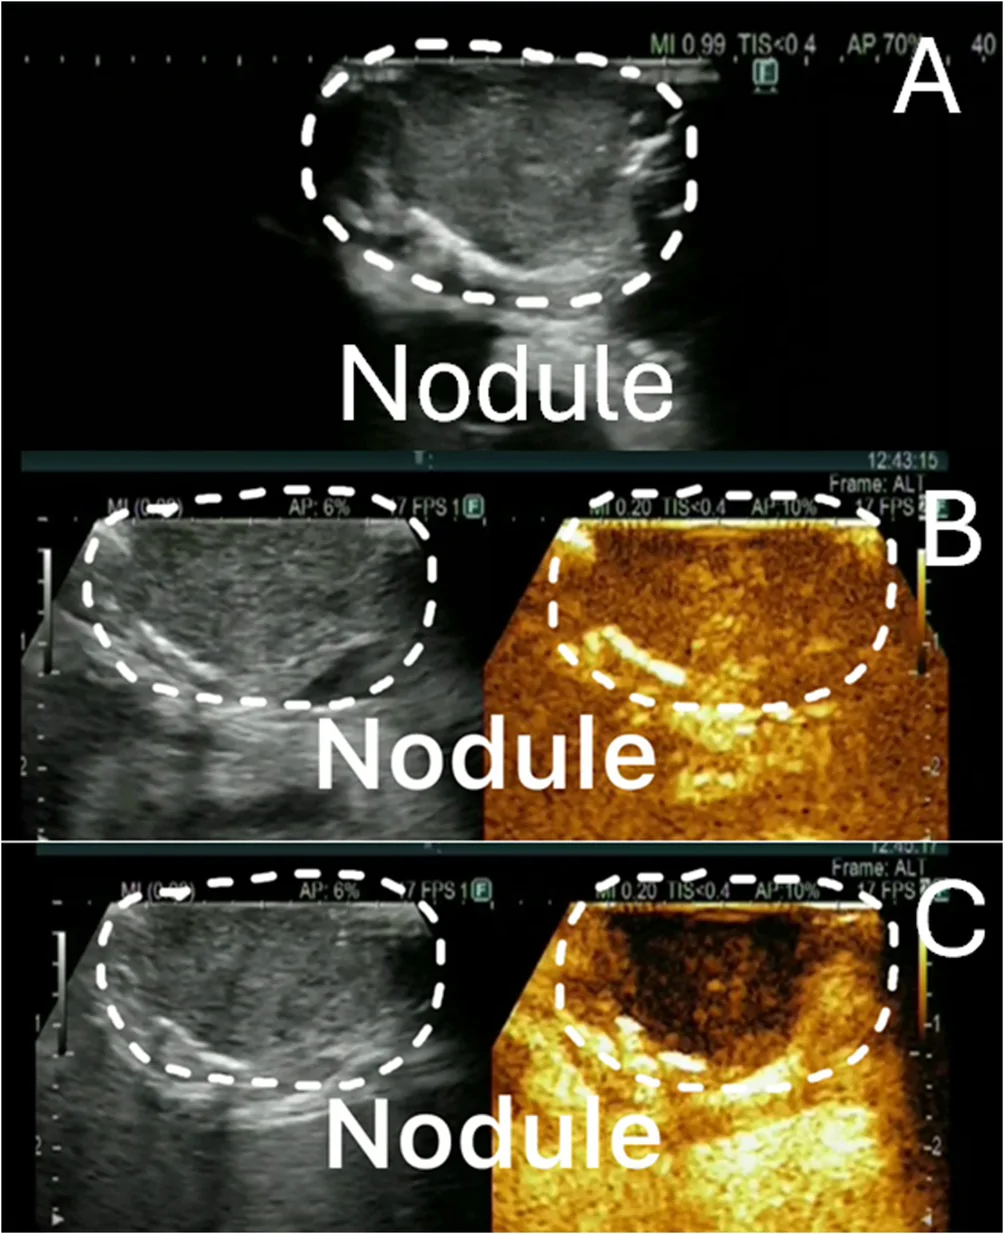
The nodule was identified as a hypoechoic lesion by ultrasound (A). After administration of contrast‐enhanced, it presented hyper‐enhancement during wash‐in phase (B) and hypo‐enhancement during wash‐out phase (C).
